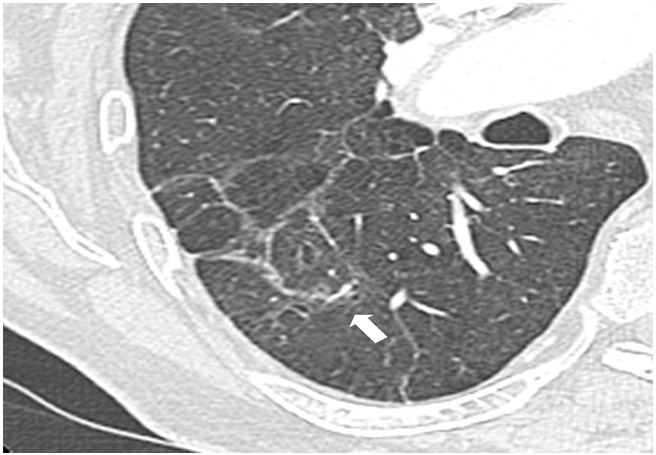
Third post-contrast CT scan, with axial reformation, performed 3 months later showing total disappearance of the mass (large arrow). There was a complete disappearance of pulmonary emboli (not shown)
